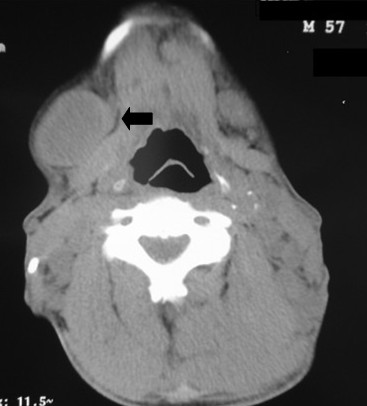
The computed tomography scan of the neck shows an enlarged right submaxillary lymph node.
